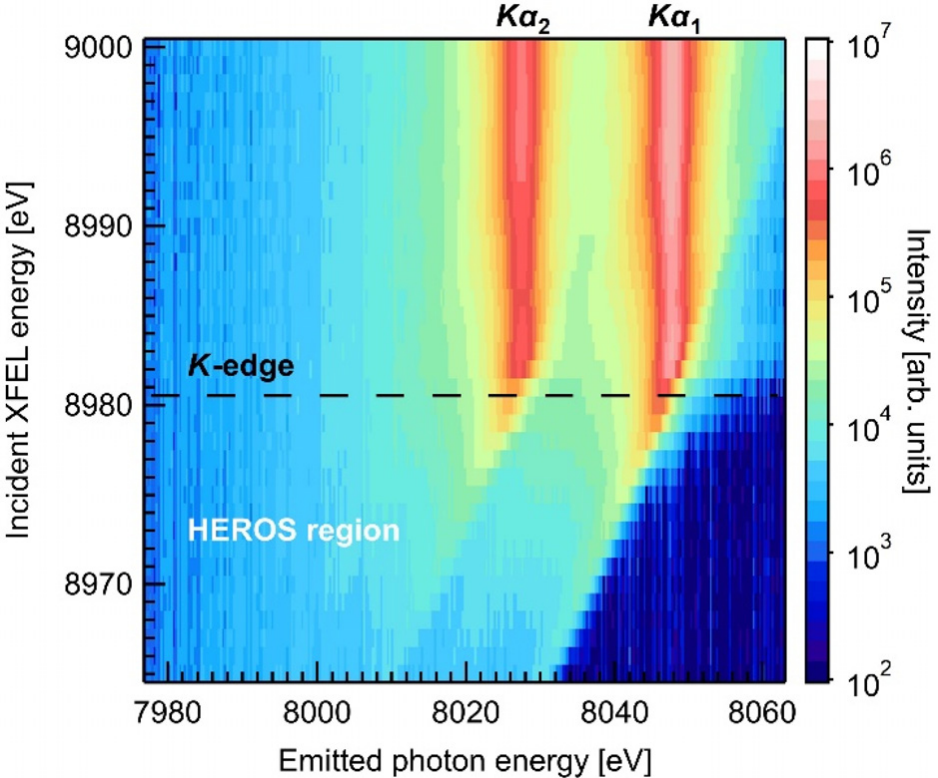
The intensity map of emitted x-ray photons from 5-*μ*m-thick Cu foils.
